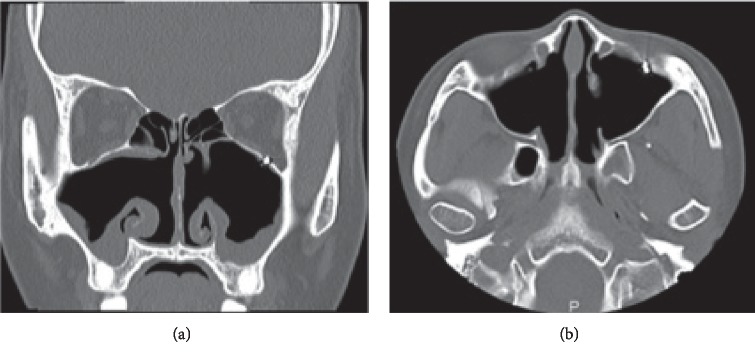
Computed tomography (CT) image (postoperation). Postoperative (a) coronal and (b) axial CT scans show the both sides of nasolacrimal duct and inferior turbinate were preserved. Also, the coil in the left infraorbital artery was seen in infraorbital canal.
